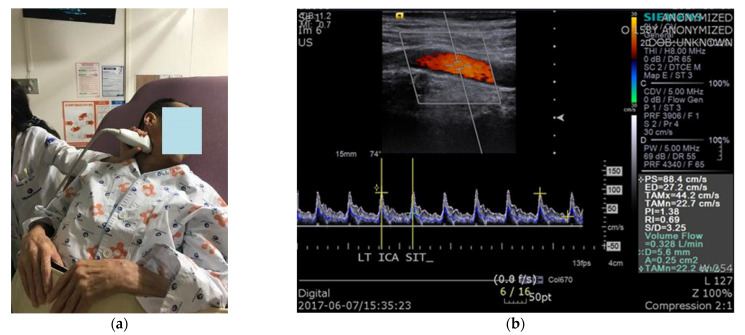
Carotid duplex sonography: (a) The position of the patient and measurements of cerebral blood flow using carotid duplex ultrasonography; (b) The value of interest in the test was volume flow.
